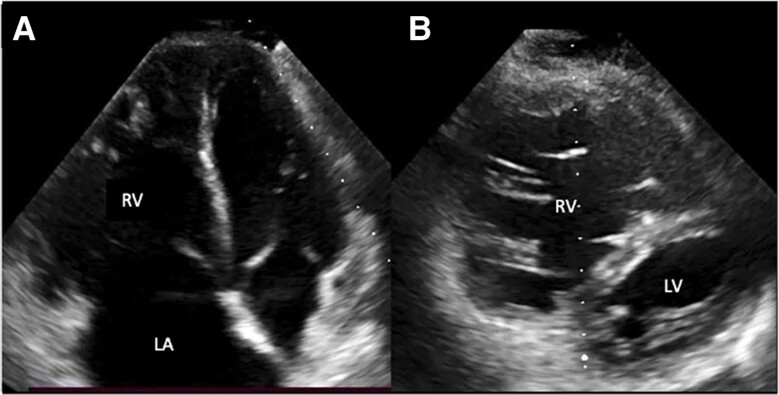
Transthoracic echocardiography. (*A*) Apical four-chamber view shows marked dilation of the right atrium (LA) and right ventricle (RV). (*B*) Short-axis view shows dilation of the right ventricle and flattening of the interventricular septum in systole.
